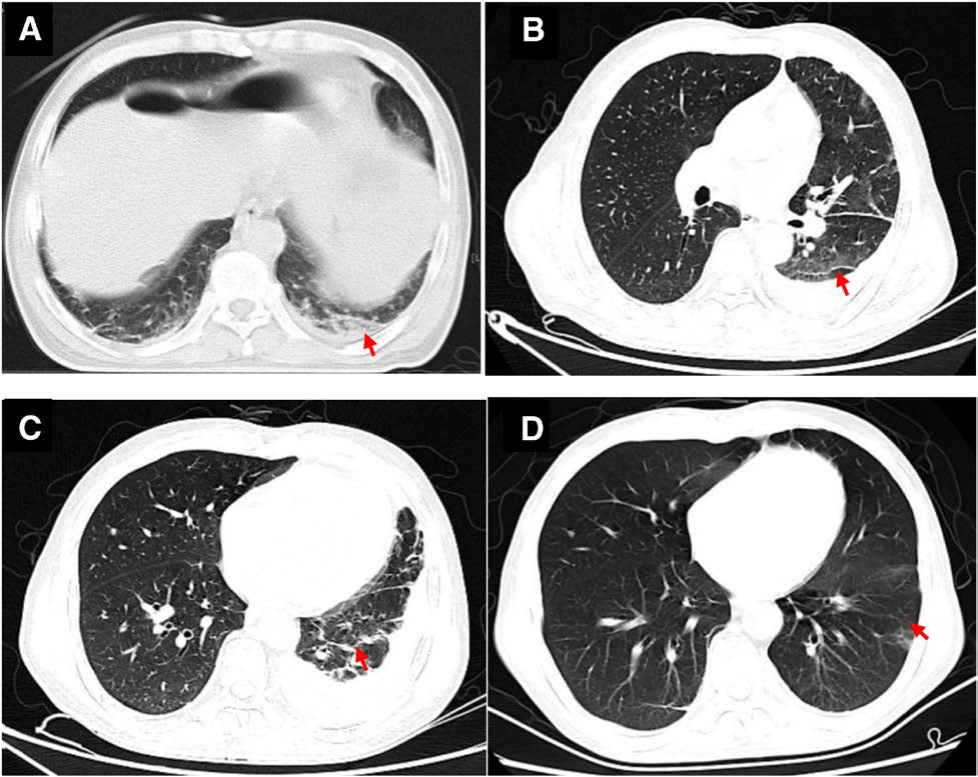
Computed tomography of lungs. Inflammatory exudate in both lower lobes of the lungs (A, preoperative);Multiple flaky and patchy shadows in the apical right lung, lower lobe of both lungs and upper lobe of the left lung (B and C, preoperatively); patchy ground-glass opacity under the left lung subpleural (D, postoperative).
